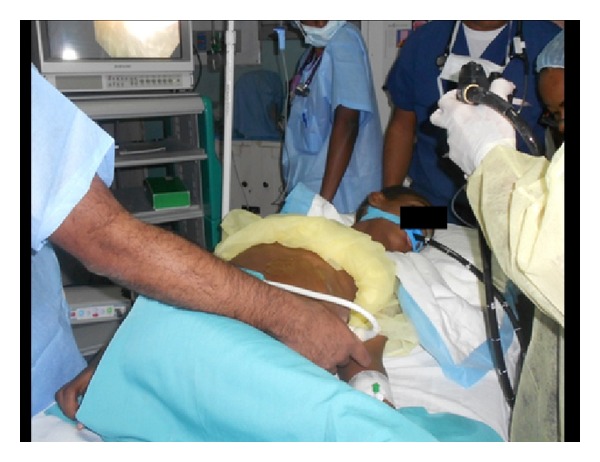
Gastroscope advanced into stomach to identify the area of bulging at the posterior gastric wall. Simultaneous transabdominal ultrasound being performed to guide the endoscopist to the ideal area for puncture.
